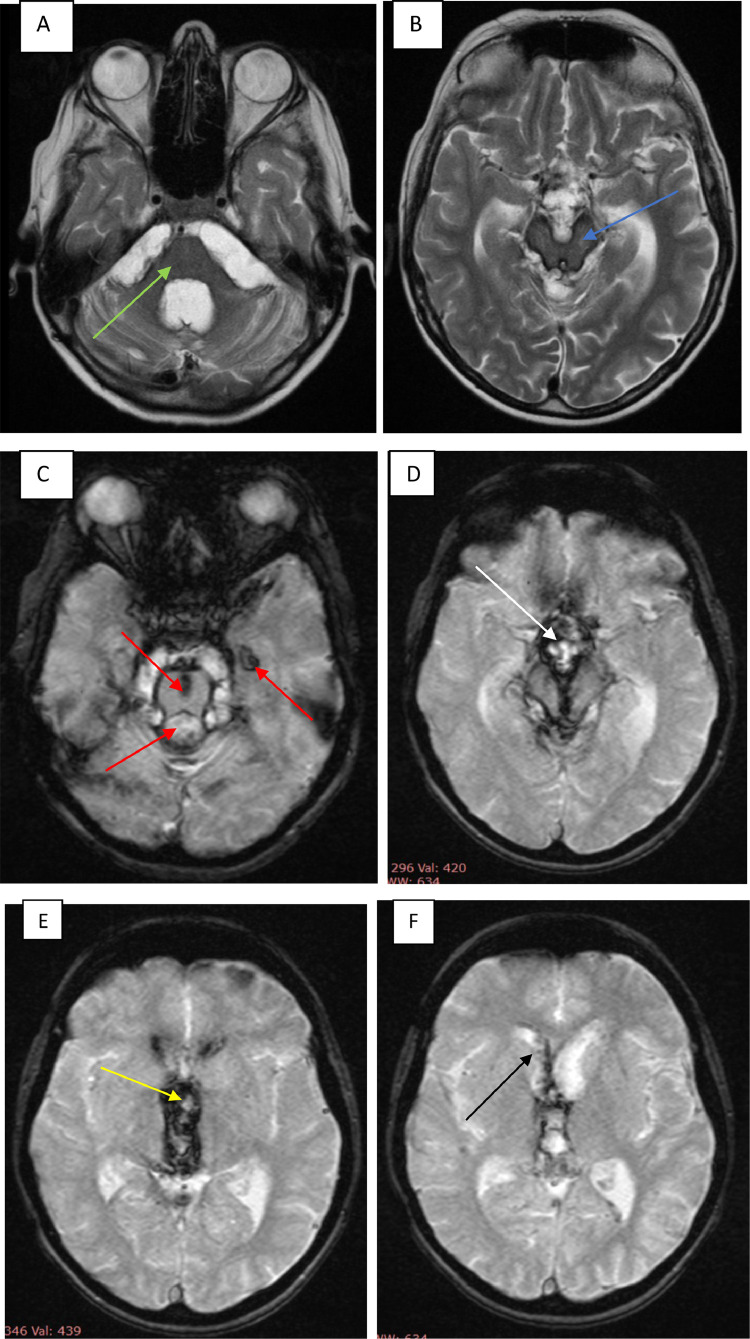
Typical magnetic resonance imaging (MRI) findings in superficial intraventricular surface siderosis. T2-weighted axial images present hemosiderindeposits along the cerebellar peduncles, around the IV ventricle (A) (green arrow) mesencephalon) (B) (blue arrow). T2-weighted images GRE presents hemosiderin deposits along the peripontine cistern, pons, Sylvi Aquedactus, tentorium, temporal horn of the left lateral ventricle (C) (red arrows), mesencephalon, suprasellar pons cistern (D) (white arrow), III ventricle (E) (yellow arrow), and frontal horns (F) (black arrow).
